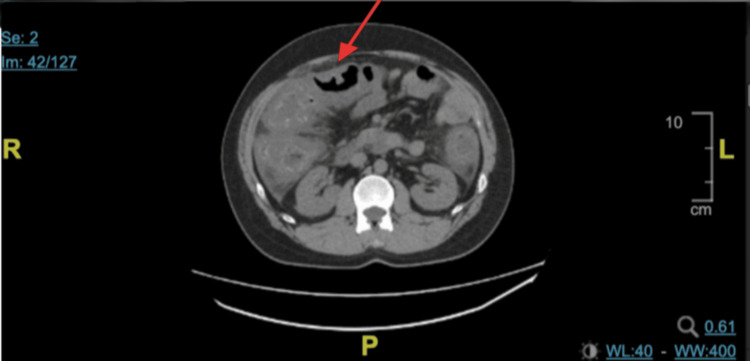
CT scan of the abdomen without contrast CT scan of the abdomen without contrast showed severely thickened and inflamed colonic wall consistent with pancolitis (red arrow). CT: computed tomography
